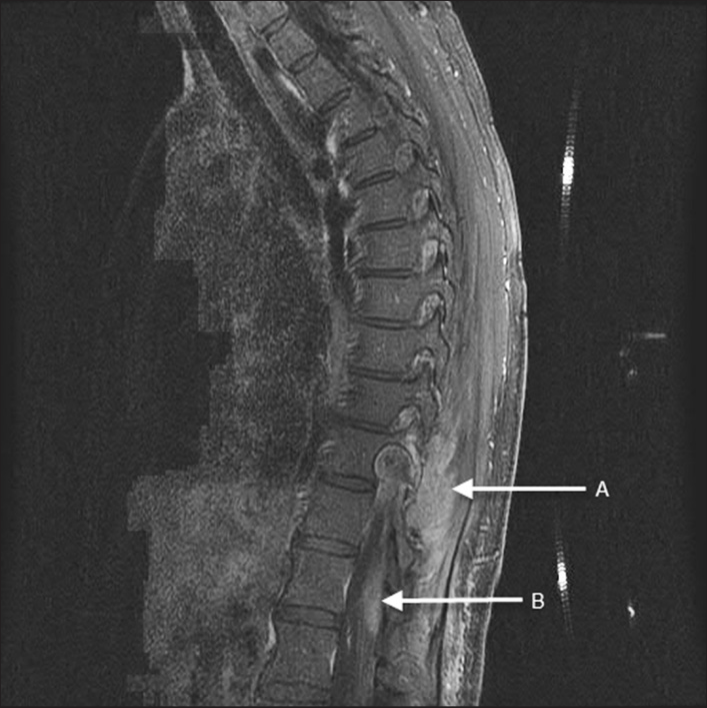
Preoperative T1-sagittal MRI with contrast shows (A) a paraspinal mass extending through the foramen as well as (B) leptomeningeal spread
